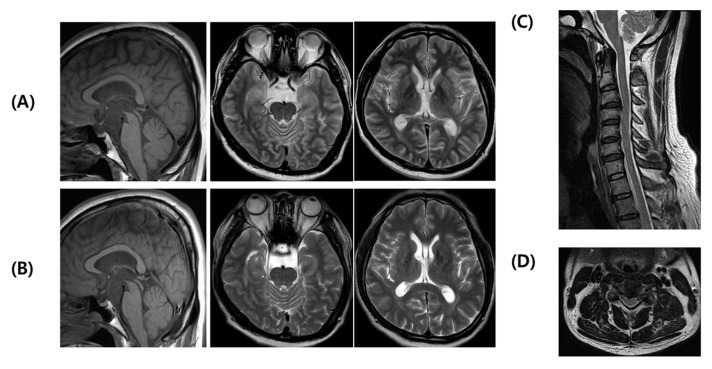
MRI images of the patient with Val1335IIe. (A) 3 months after onset and (B) 8 months after onset of brain MRI. Sagittal T1-weighted image showing an arachnoid cyst in the suprasellar-interpeduncular cistern displacing the third ventricular flow upward ((A)-Left). T2-weighted images in the pons ((A)-Middle) and around thalami ((A)-Right) exhibit no evidence of cerebellar atrophy. The serial MRIs show no change of the cerebellar peduncles ((B)-Left), pons ((B)-Middle), and thalami ((B)-Right). (C,D) The MRI of cervical spine. The sagittal and axial T2-weighted images presenting central canal stenosis (prominent at C3/4 level) without atrophy of the cervical spinal cord.
